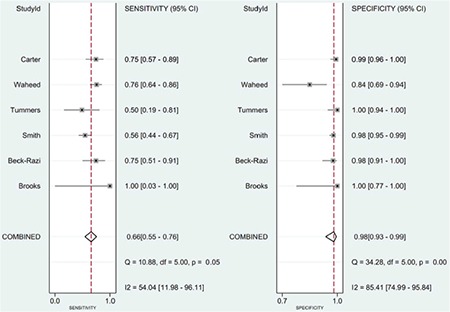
Summary of sensitivity and specificity of focused assessment with sonography in trauma for assessing injury.
